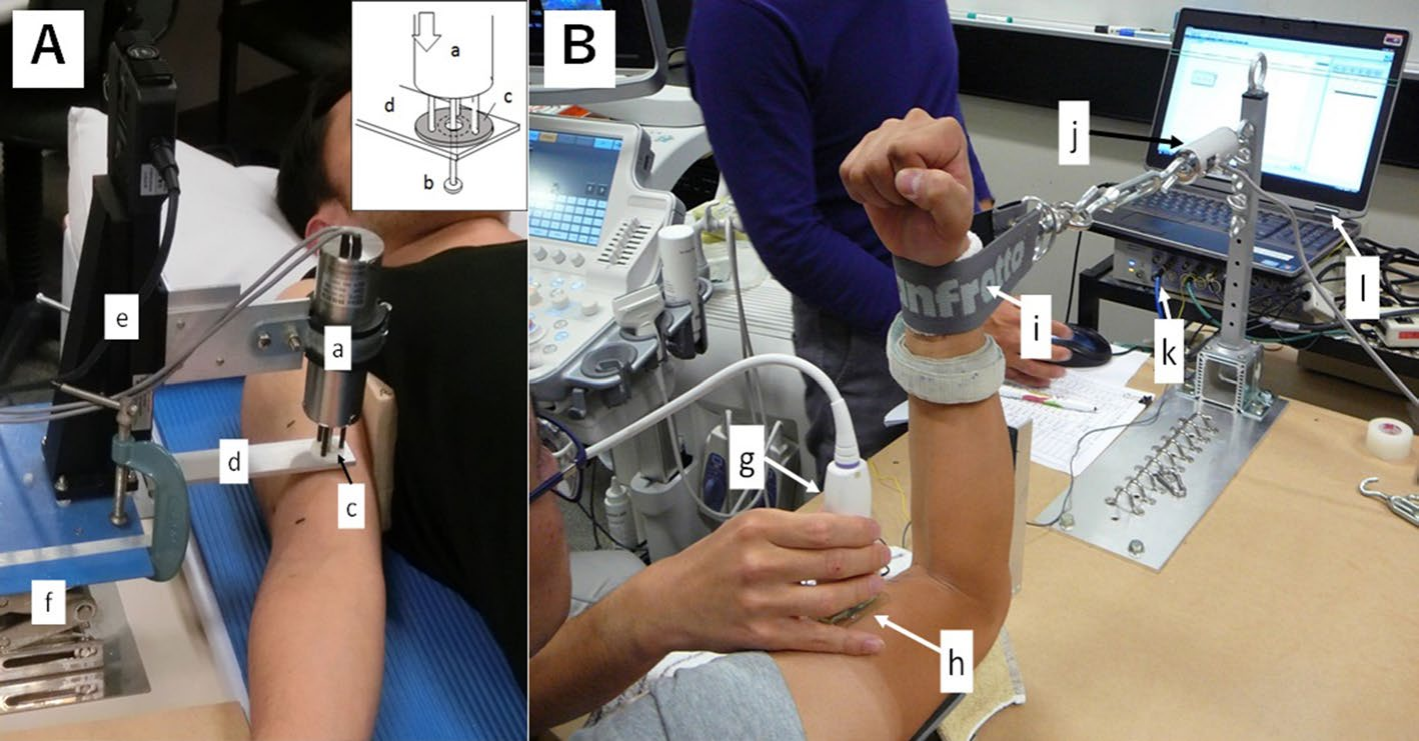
Set-up for the push-in meter (PM) assessment of muscle hardness in resting condition (A) and for the ultrasound strain elastography (USE) assessment of muscle hardness in contracting condition at 90° elbow angle as an example (B). (A) The inset shows the detail of the probe of PM. The cylindrical body of PM (a) has an un-movable probe (ϕ = 10 mm, length = 30 mm) with a force transducer (b) and a movable sensor plate for displacement detection (c). PM was attached to a z-axis stage (e) which controlled by the stage control unit. The stage was set on a jack (f), and PM was set on a stopping bar (d) fixed to the jack. A hole on the stopping bar only allowed the probe to enter. Moving the stage caused the probe to depress and push the underlying tissue while the sensor plate was pushed back by the stopping bar, detecting tissue displacement. In this system, 20-mm pushing into the tissue was performed from the skin surface. Each participant lied on his back on the table and the elbow kept at an elbow extended position (0°). (B) A probe (g) of USE is placed on the muscle belly with an acoustic coupler (h) in between. The investigator manually compressed the muscle by the probe. A strap (i) is attached to the wrist that is connected to a force transducer (j) with a turnbuckle. The force transducer is connected to a data acquisition system (PowerLab) (k) via a computer (l). Each participant was provided the visual feedback of their performed force on a computer screen simultaneously. The same set-up of a participant as that is shown in A was used for the USE measure for the resting muscle, and the same set-up of a participant as that is shown in B was used for the PM measure too.
